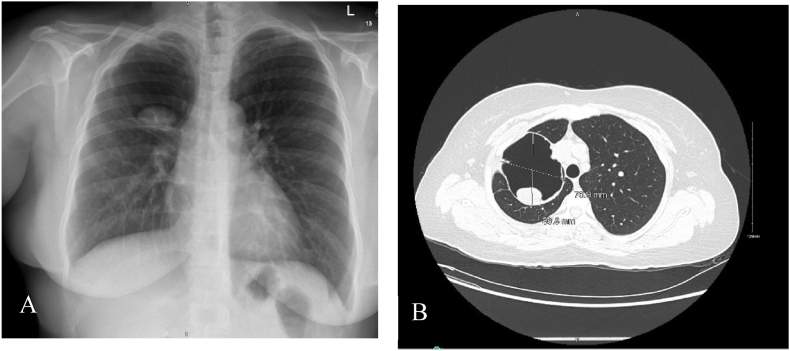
(A) Chest X-Ray with large cyst in the right upper lobe. (B) CT Scan shows the cyst with persistent internal solid component and new adjacent air fluid level and internal air foci.
